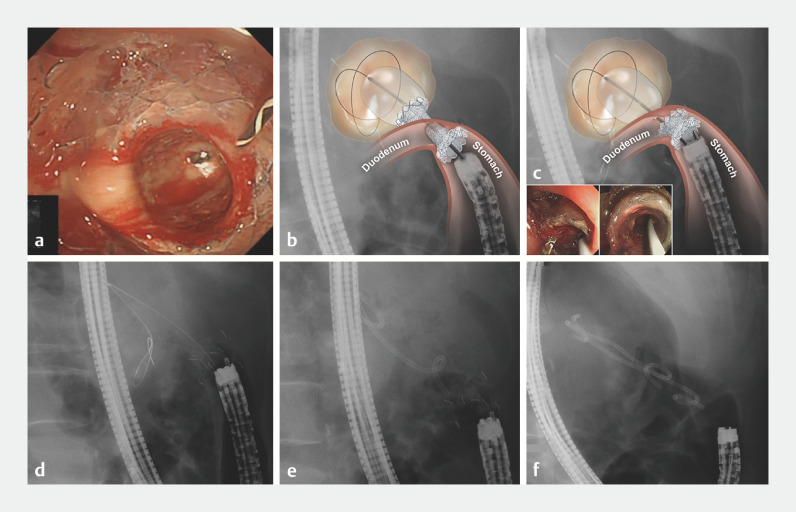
Retrieval balloon catheter-assisted rescue technique for misplacement of a lumen-apposing metal stent during endoscopic ultrasound-guided transduodenal walled-off necrosis drainage.
